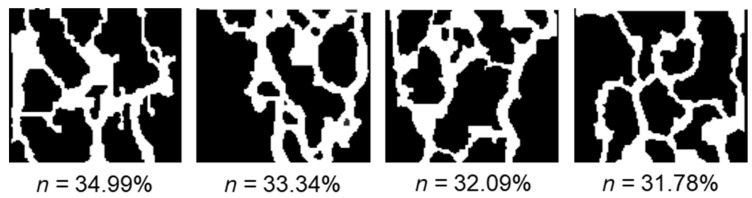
CT images of the GRS with different porosities.
